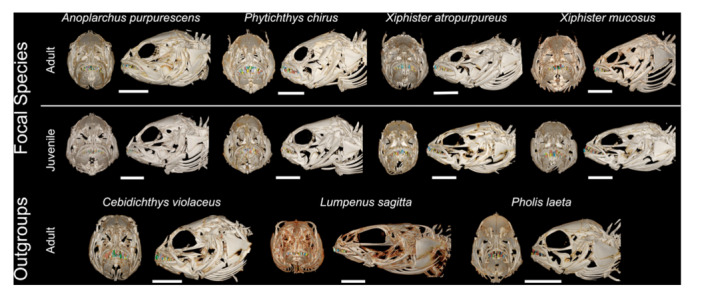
Micro CT volume renderings of skull morphology. Dentition segmentations overlaid, multicoloured. Adult fish scale bars = 5 mm, juvenile = 2.5 mm.
